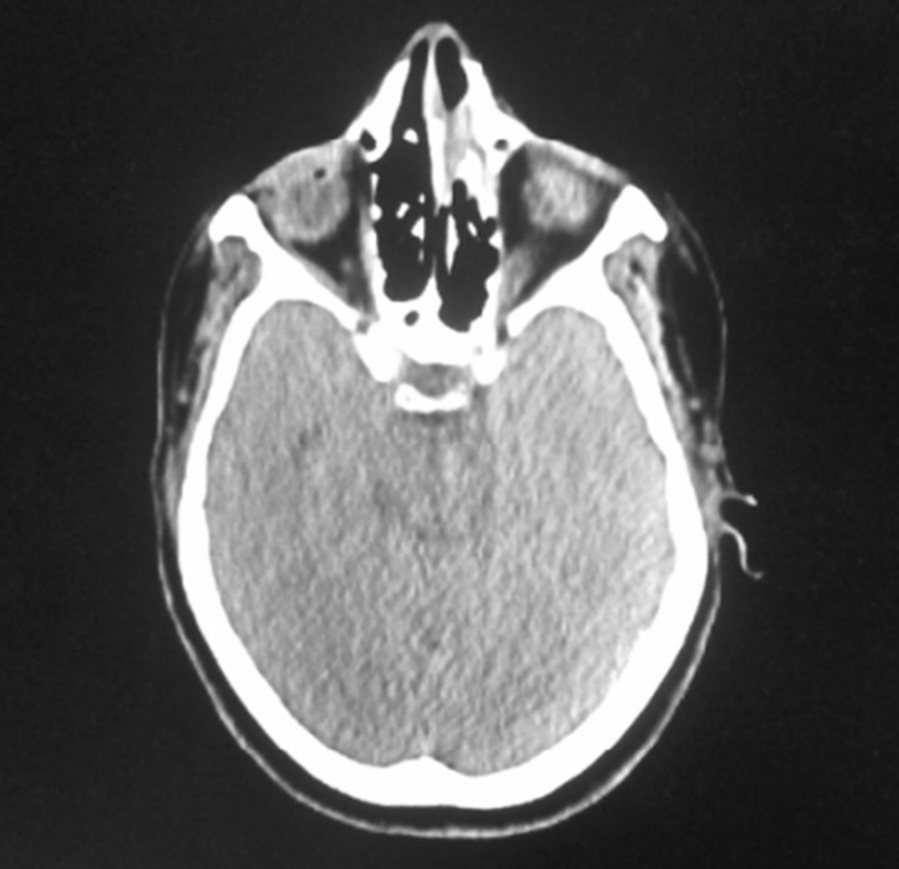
Axial computed tomography (CT) of evaluation showed a near complete response in the nasal cavity
